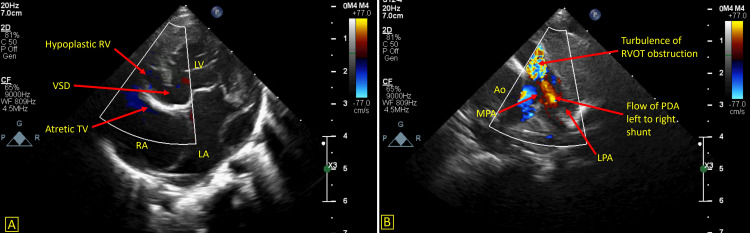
A: Showing an apical four chamber view. B: Color Doppler of the parasternal short axis view showing a patent ductus arteriosus with left to right shunt. LA – Left atrium, LV – Left ventricle, RA – Right atrium, RV – Right ventricle, RVOT – RV outflow tract, Ao – Aorta, TV – Tricuspid valve, VSD – Ventricular septal defect, PDA – Patent ductus arteriosus, MPA – Main pulmonary artery, LPA – Left pulmonary artery.
